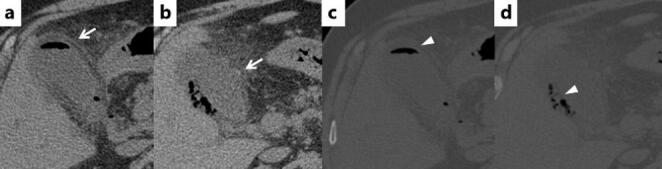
Emphysematous cholecystitis. a–b CT, c–d CT (bone window). A man in his 70s with type 2 DM presented with a chief complaint of abdominal pain. CT (a–d) demonstrates gallbladder wall thickening, pericholecystic fluid, and inflammatory fat stranding (arrow). Emphysematous cholecystitis was suspected because of the presence of air along the gallbladder wall (arrowhead). Cholecystectomy was performed, and the patient was diagnosed with emphysematous cholecystitis. Abbreviations: CT, computed tomography; DM, diabetes mellitus
